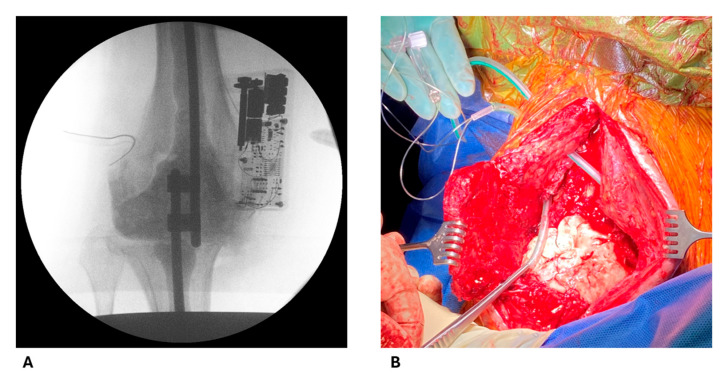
Spacer and catheter placement. (A) Postoperative X-ray photograph showing the metal rods implanted into the tibia and femur and connected at the previous knee joint line. The redon tube with the microdialysis catheter is seen on the left and the pump connected to the catheter on the right. (B) The modeling of the spacer has been concluded. The drainage tube containing the microdialysis catheter has been inserted in the lateral compartment of the knee joint (tube held with forceps) and will be placed laterally on the cement. The inlet and outlet tube (held in hand) will be connected accordingly to the microdialysis pump and microvial. The second drainage tube located further cranial is used as a redon drain.
